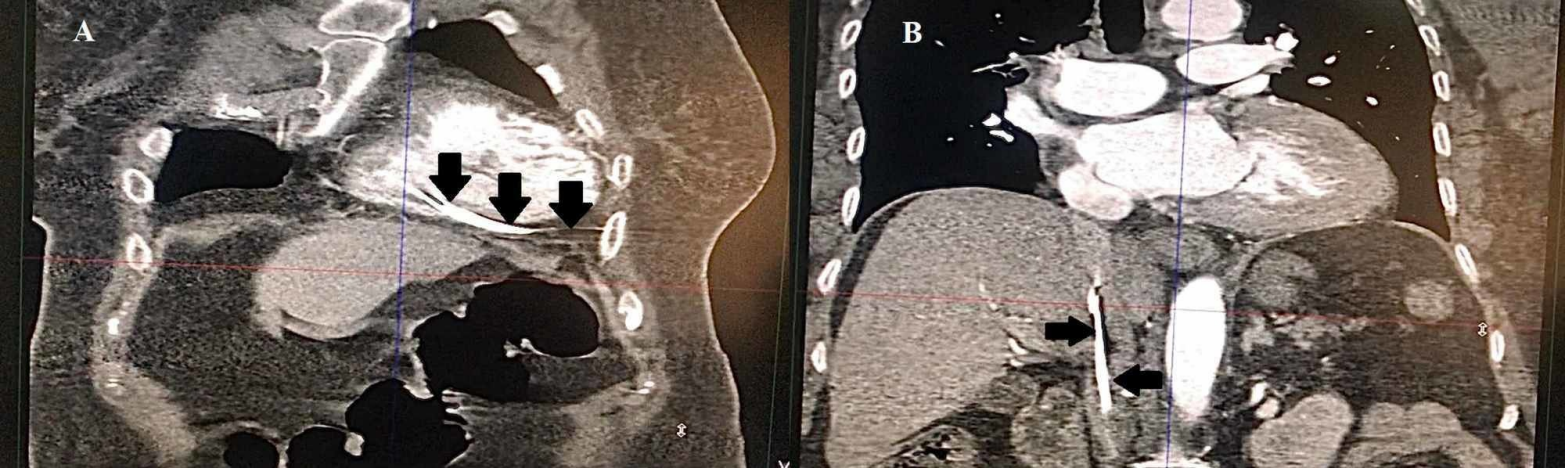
(A, B) Frontal plane of CT scan chest and abdomen showing the pacing wire (black arrows) coming via the inferior vena cava and going through the right ventricle into the thorax and abdominal cavity.
